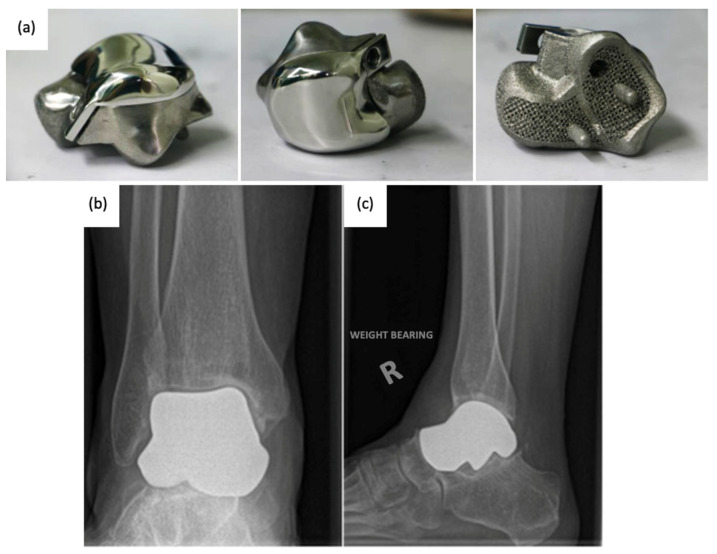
(a) 3D-printed third-generation total talus prosthesis and post-operative X-rays of implanted 3D-printed third-generation TTA prosthesis, (b) anterior–posterior, and (c) lateral views [46,49].
